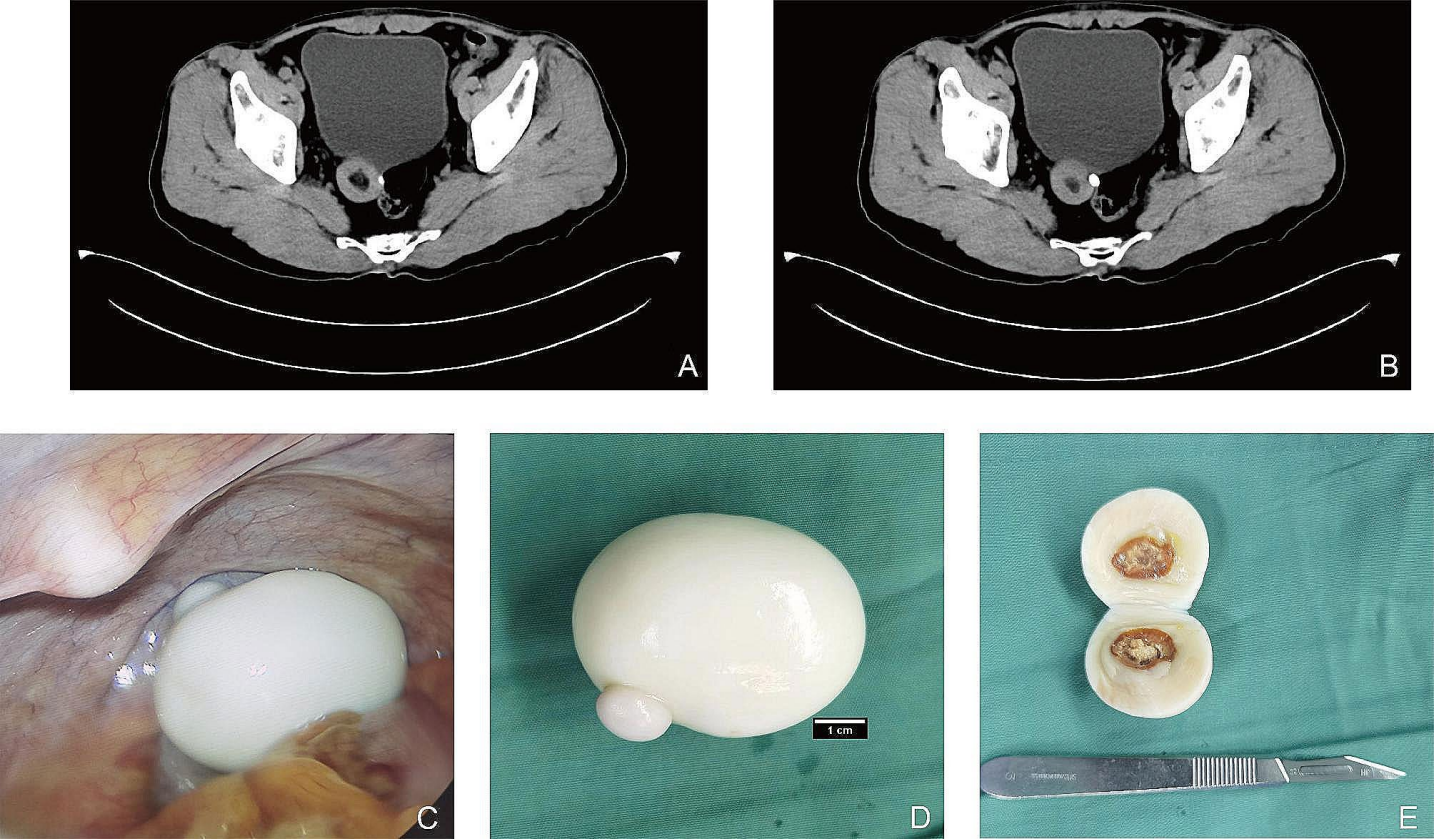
A&B: Abdominal CT showed a 4*3.8 cm mass in the right space between bladder and rectum; C: Morphology of the lesion under laparoscopy. D: The lesion is a white oval mass with a size of about 4.5*4*3 cm, which is slippery, tough, no adhesion with the surrounding tissue. E: The lesion had a calcified center
